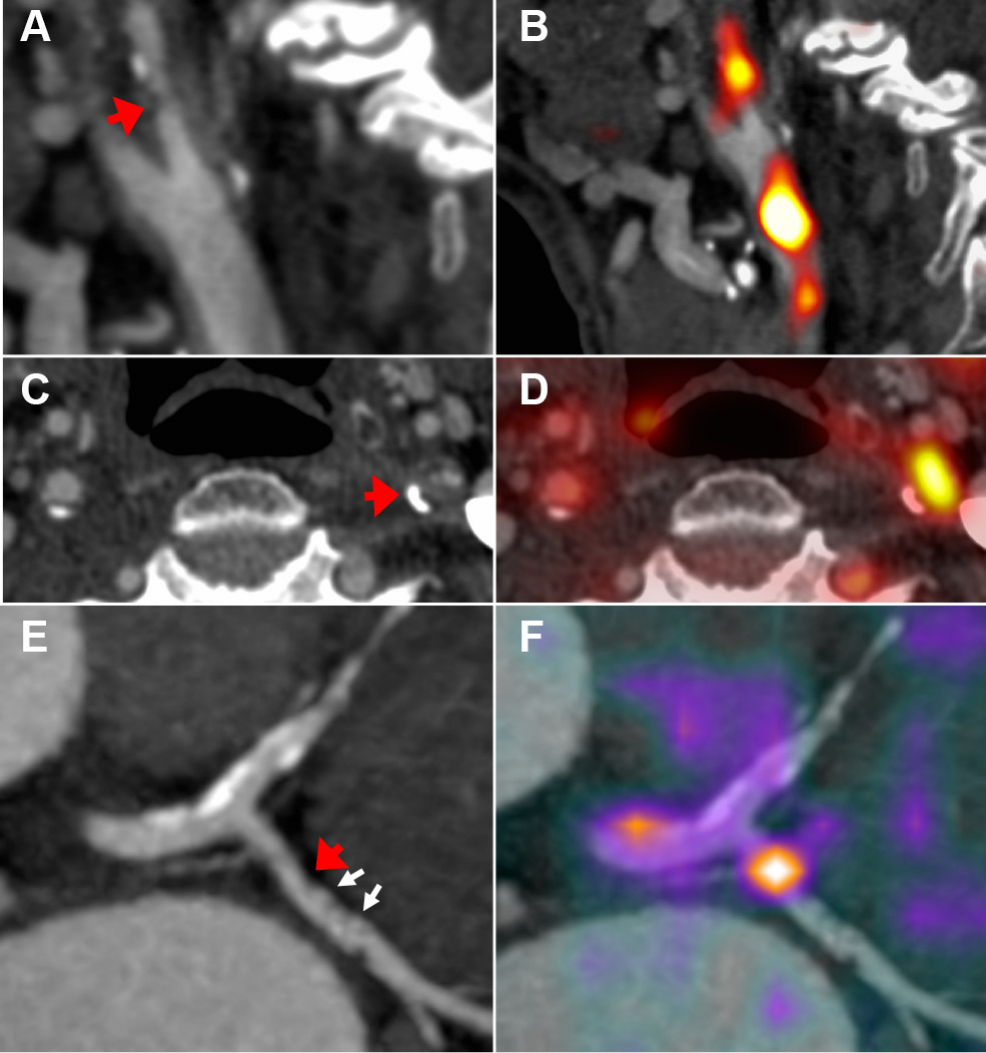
Positron emission tomography (PET) inflammation imaging. Computed tomographic (CT) angiography of symptomatic left internal carotid stenosis (arrows); sagittal (A) and axial (C) views. B, D, Fused ^18^F-fluorodeoxyglucose (FDG) PET–CT demonstrates high uptake relating to the symptomatic carotid plaque. E, Coronary CT angiogram of left circumflex coronary artery lesion (red arrow) with spotty calcification (white arrows); F, fused ^68^Ga-DOTATATE PET–CT demonstrates high signal in relation to the inflamed coronary plaque.
